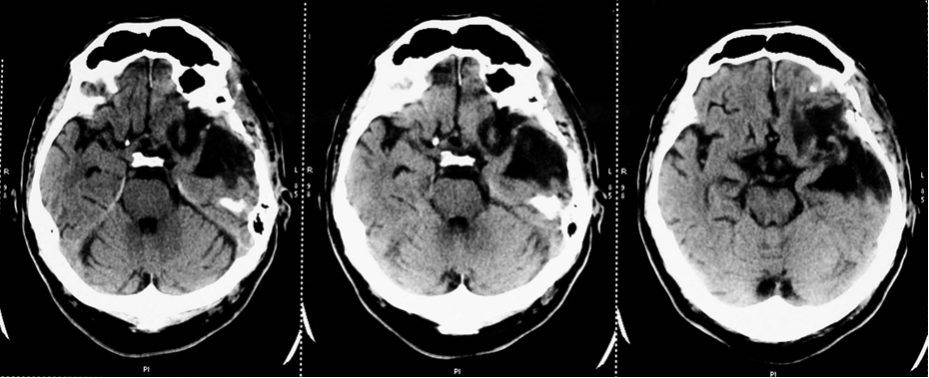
Preoperative computed tomography (CT) scan. Subdural collection with capsule organization and collateral brain edema on the side of the previous tumor is clearly visible.
